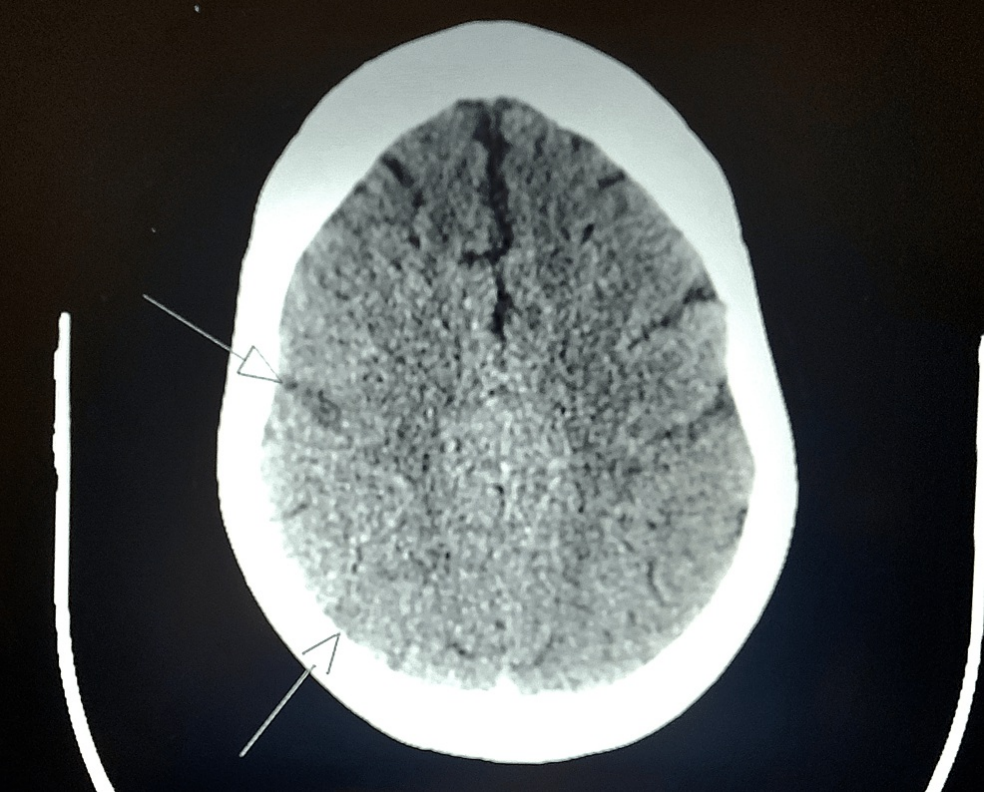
CT brain showing acute infarct in the right frontal and right parietal lobes. Arrows demonstrate two small hypodense areas indicative of evolving small acute infarcts in the right frontal and parietal lobes.
